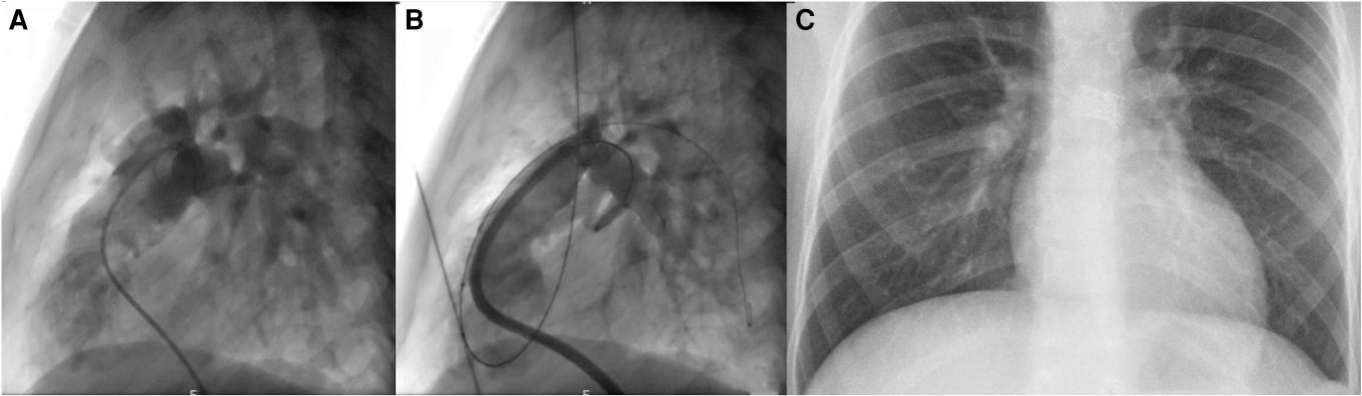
Case 1 angiograms in lateral projection. (*A*) Initial angiogram in main PA. Due to surgically augmented right ventricular outflow tract and aneurysmally dilated peripheral pulmonary arteries, the branch PAs and stents are more difficult to assess in lateral view compared to cranial projections. The lateral projection is however useful for precise balloon and stent positioning, using biplane fluoroscopy with cranial and lateral projection simultaneously. (*B*) Final angiogram in the lateral view helps to confirm absence of vascular wall complications. (*C*) Post-procedural chest X-ray confirming stable PA stents position and absence of lung parenchymal injury.
